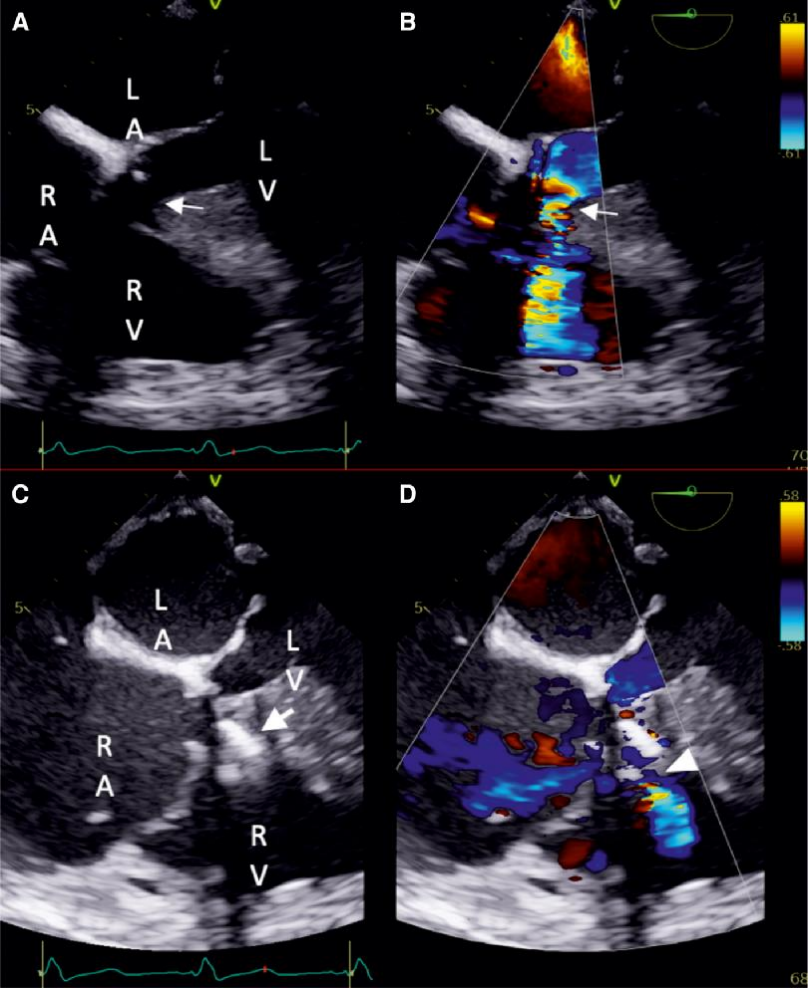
(*A*, *B*) Transoesophageal four-chamber view, 2D colour comparison showing the ventricular septal defect. Arrow: ventricular septal defect. (*C*, *D*) Transoesophageal four-chamber view, 2D colour comparison after ventricular septal defect closure. Arrow: ventricular septal defect closure device; arrowhead: residual ventricular septal defect shunt. LA, left atrium; LV, left ventricle; RA, right atrium; RV, right ventricle.
